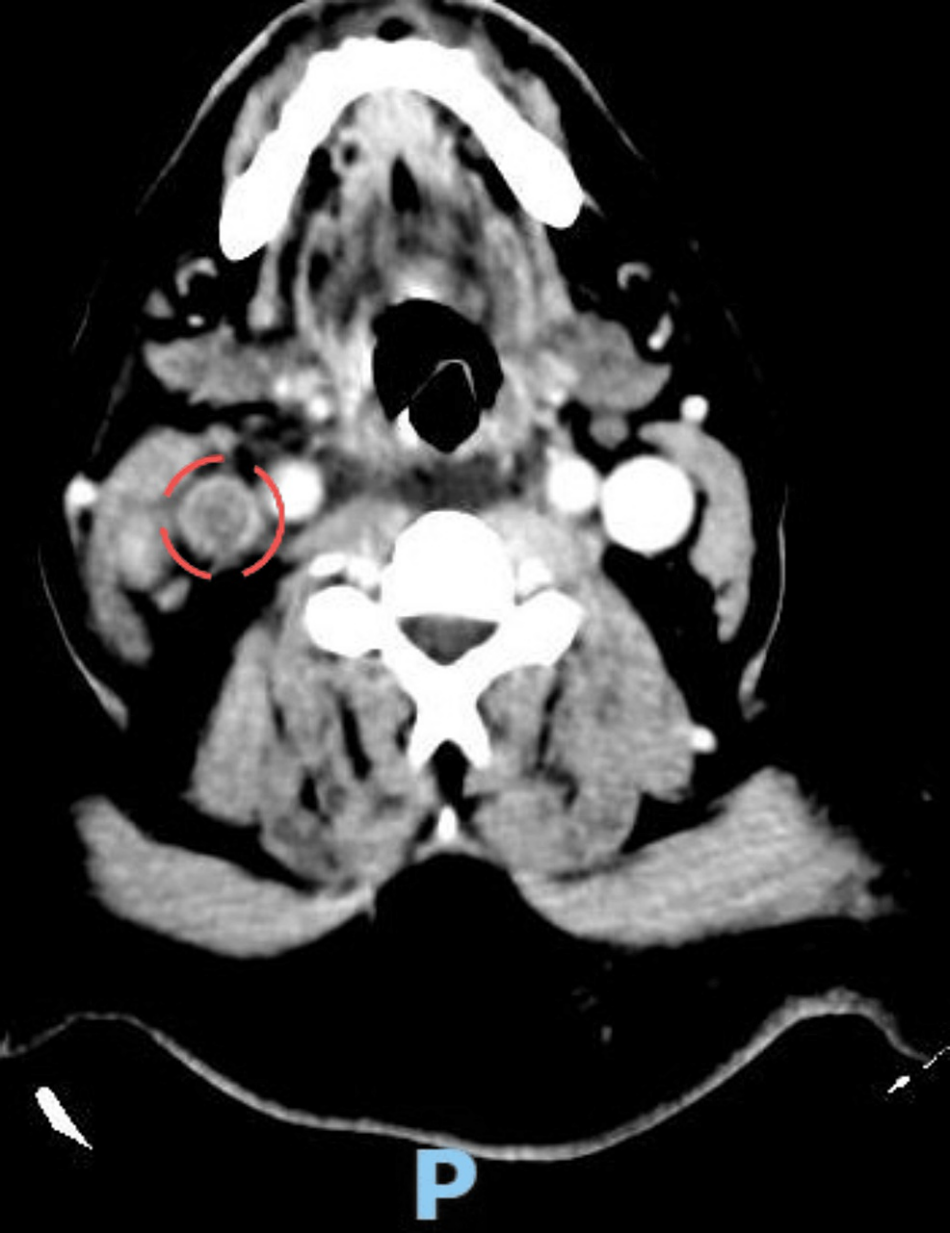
CT venography showing a filling defect of the right internal jugular vein (dashed red circle).
